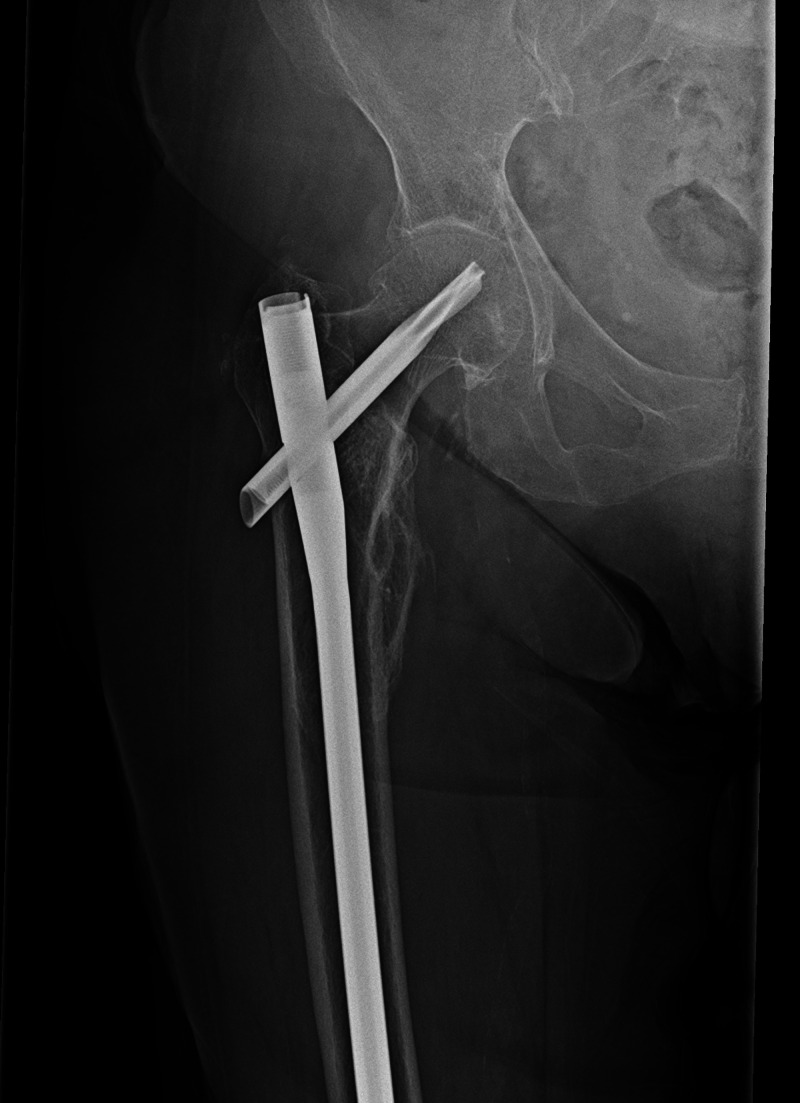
Postoperative Radiograph An anteroposterior radiograph of the right hip one-year status post cephalomedullary nail for the fracture in Figure 1.
